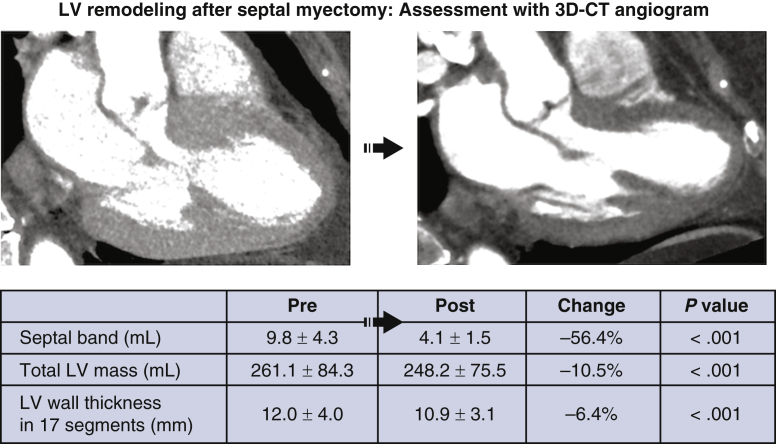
Left ventricle (*LV*) remodeling after septal myectomy: Assessment with 3-dimensional computed tomography (*3D-CT*) angiogram. The LV outflow tract obstruction has been removed and LV myocardium remodeling has occurred.
